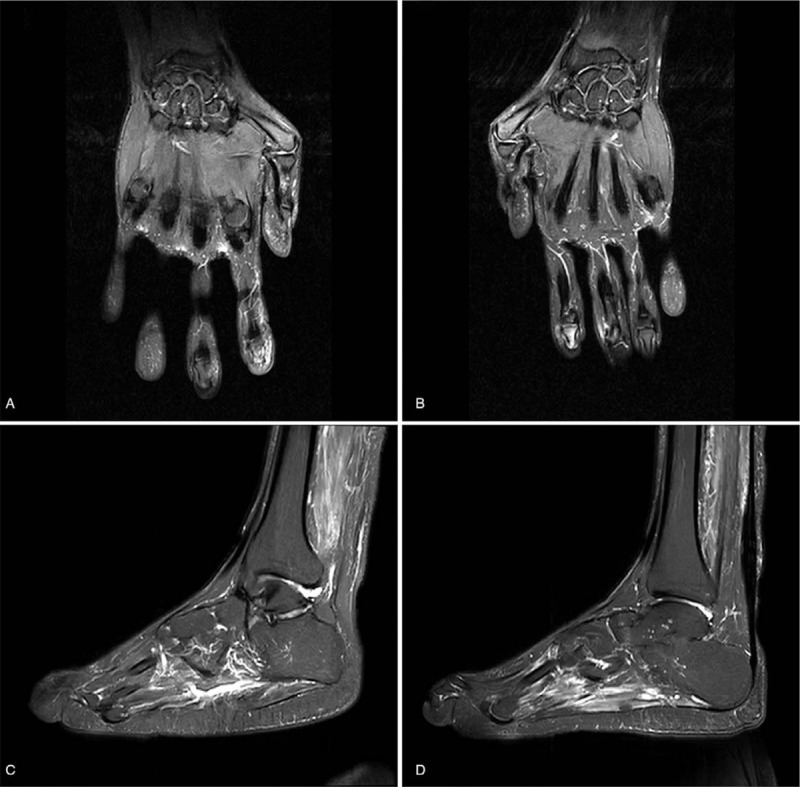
Magnetic resonance (MR) imaging T2-weighted images (T2WI) of bilateral hands and feet (a–d) revealed joint effusions, muscles and soft tissues injury in bilateral hands and feet. (a) MR imaging of left hand. (b) MR imaging of right hand. (c) MR imaging of left foot. (d) MR imaging of right foot.
